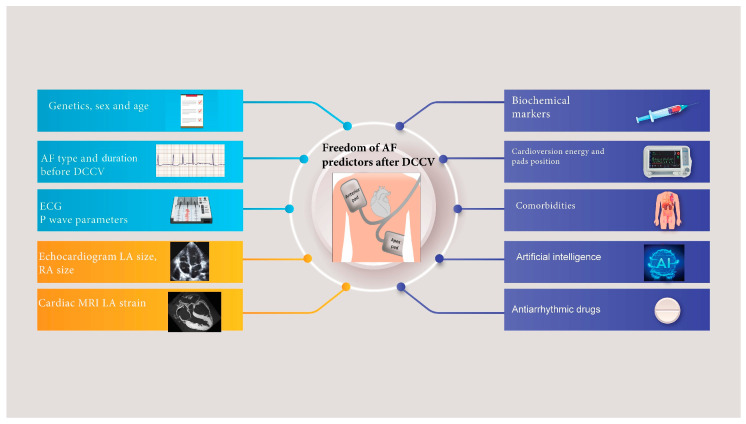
A summary of the demographic, imaging, investigative, and pharmacological factors known to predict freedom from atrial fibrillation following direct current cardioversion. DCCV: direct current cardioversion. MRI: magnetic resonance imaging. LA: left atrium. RA: right atrium. AF: atrial fibrillation.
